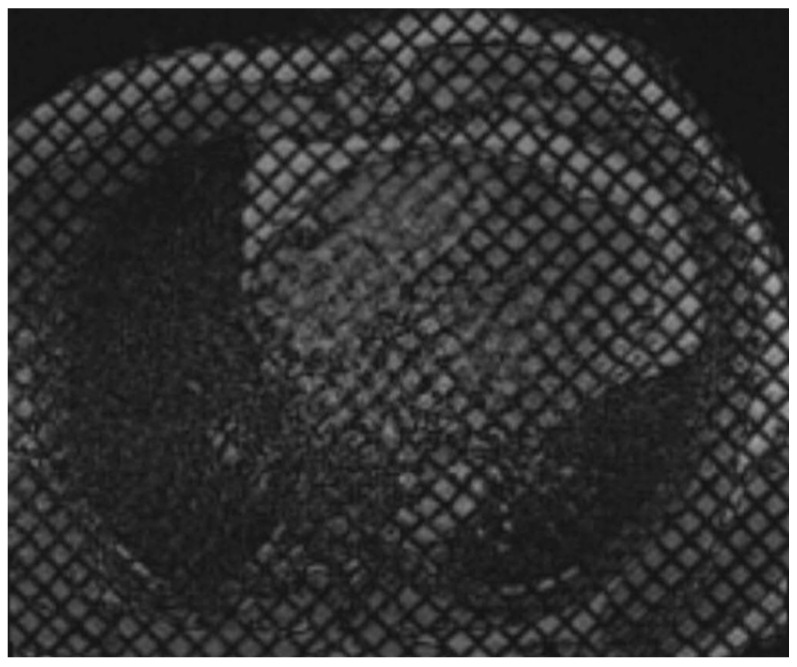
Cardiac MR Strain Evaluation. Cine horizontal long-axis grid-tagged images from a cardiac MRI. Deformation of the grid as the myocardium contracts can be used to estimate strain.
